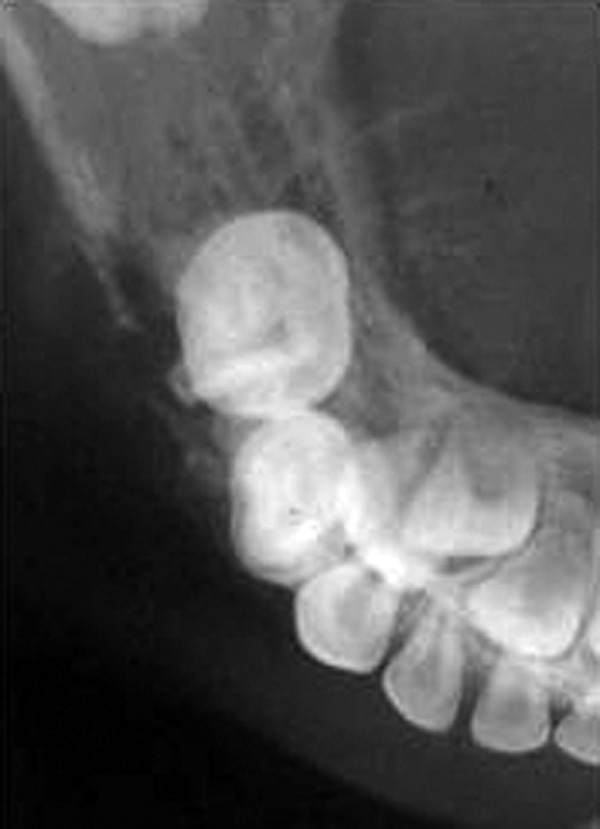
Cropped pretreatment occlusal radiograph shows radiolucent lytic lesion with cortical erosion and bony spiculations
